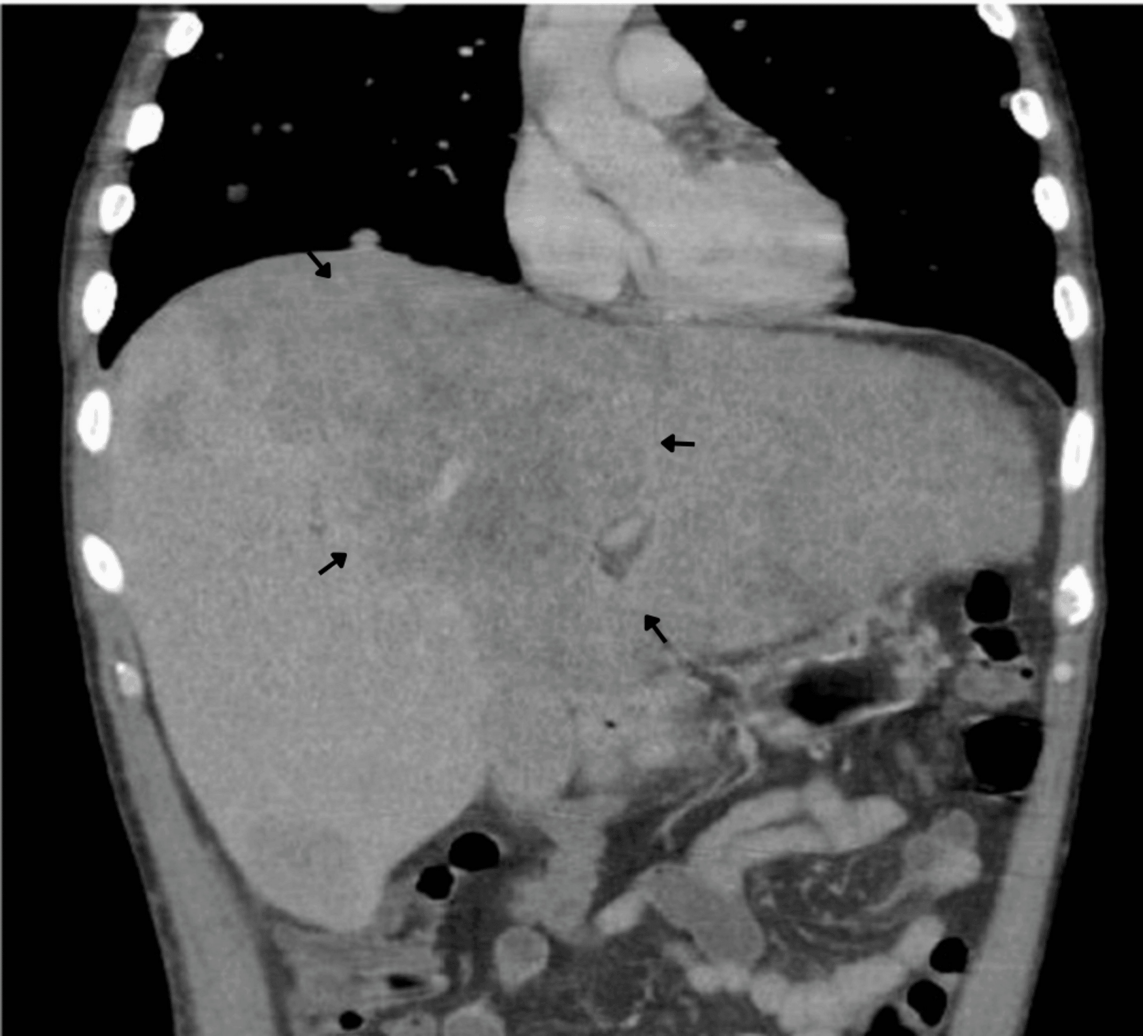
Contrast-enhanced CT of the abdomen, coronal plane. Coronal CT image reveals hepatomegaly with diffuse infiltrative involvement of both hepatic lobes. A large coalescent mass replaces most of the left hepatic lobe, findings consistent with extensive hepatic metastases. Arrows delineate the coalescent metastatic hepatic mass. CT: computed tomography
